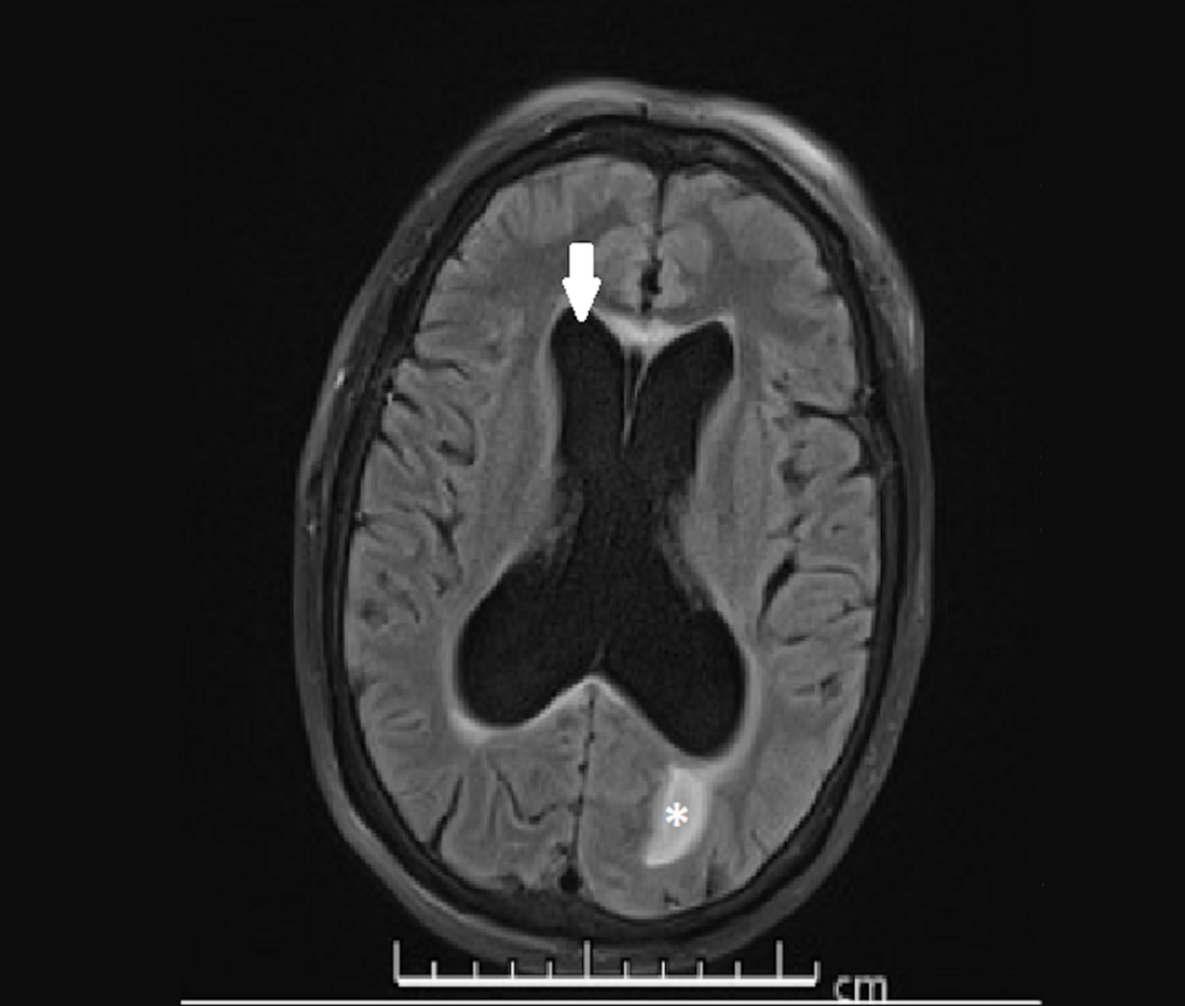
Obstructive hydrocephalus, magnetic resonance imaging (T2 FLAIR sequence). Note enlarged lateral and third ventricles (arrow), with associated transependymal flow of cerebrospinal fluid (asterisk) suggesting acuity of process.
